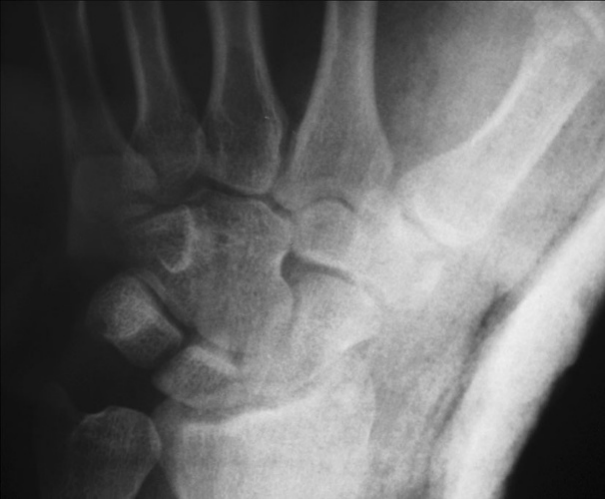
Postreduction radiograph of the carpometacarpal (CMC) joint showing adequate reduction and a congruent joint
